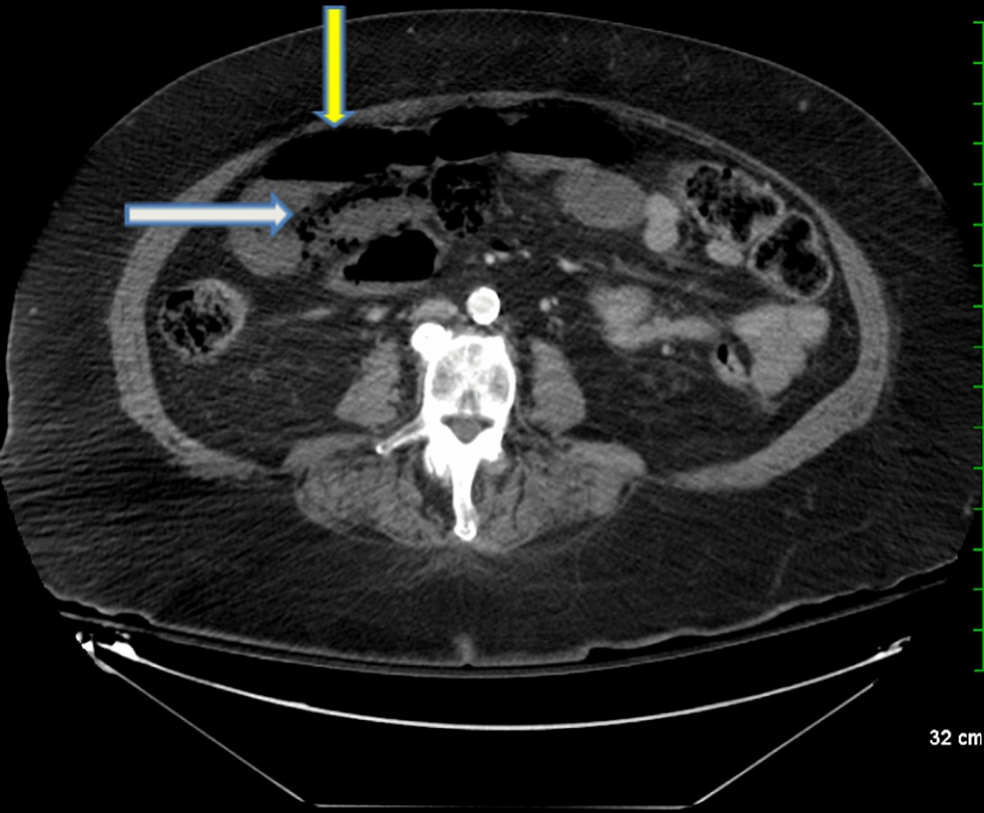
Axial view of CT demonstrating pneumatosis (white arrowhead) in a bowel loop (white arrowhead) and dilated loops of bowel (yellow arrow)
